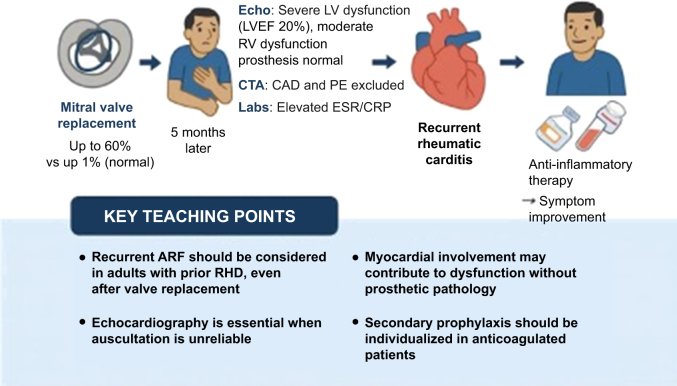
Clinical Course of a 44-Year-Old Man With Prior Rheumatic Mitral Stenosis Who Underwent Mechanical Mitral Valve Replacement and Later Developed Recurrent Rheumatic Carditis The patient presented 5 months postoperatively with new-onset dyspnea, orthopnea, and peripheral edema. Echocardiography revealed severe left ventricular (LV) systolic dysfunction (left ventricular ejection fraction [LVEF] 20%) and moderate right ventricular (RV) dysfunction, with normally functioning prosthesis. Coronary and pulmonary computed tomography angiography (CTA) excluded obstructive coronary artery disease (CAD) and pulmonary embolism (PE), and laboratory testing demonstrated elevated inflammatory markers. Recurrent rheumatic carditis was diagnosed and managed with anti-inflammatory therapy and guideline-directed medical therapy for heart failure, resulting in symptomatic improvement. Key teaching points emphasize the importance of considering recurrent acute rheumatic fever (ARF) in adults after valve replacement, the pivotal role of echocardiography, recognition of possible myocardial involvement, and the need for individualized secondary prophylaxis in patients receiving anticoagulant therapy. CRP = C-reactive protein; ESR = erythrocyte sedimentation rate; RHD = rheumatic heart disease.
